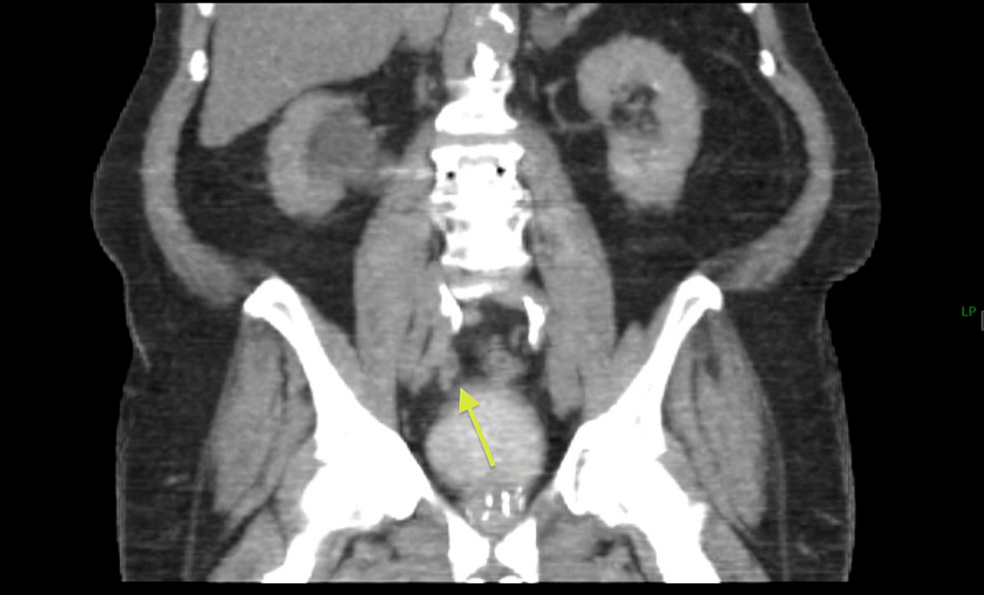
Case II CT urogram The arrow here shows narrowing in the distal right ureter with proximal hydroureteronephrosis
